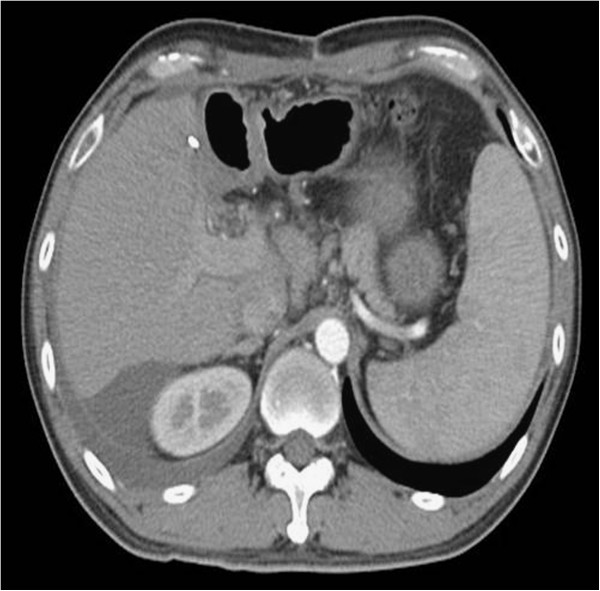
Computed tomography (CT) scan image after surgery.
